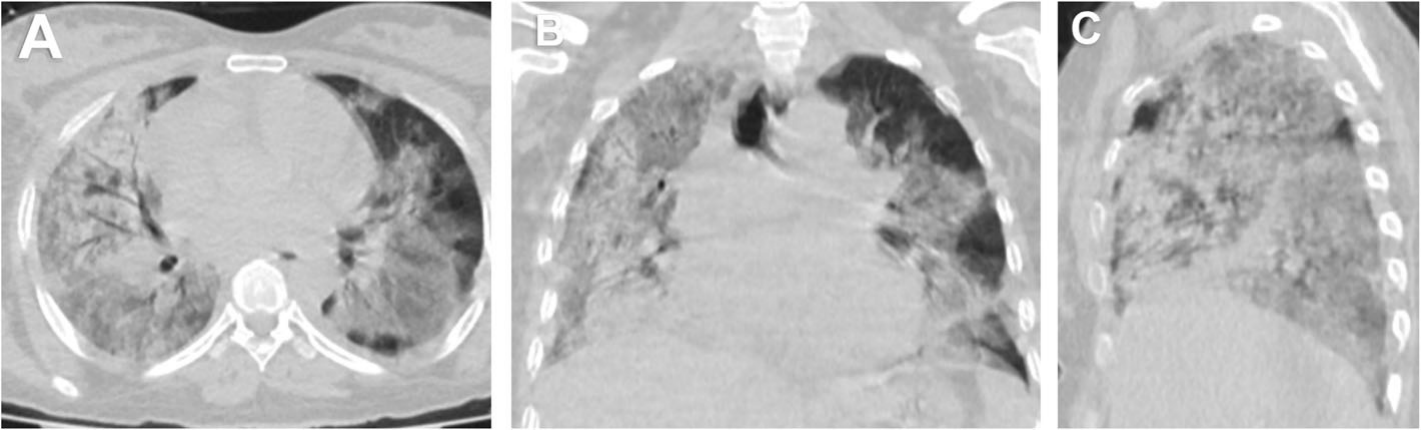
A 30-year-old female patient. A axial, B coronal, and C sagittal chest CT images show bilateral diffuse ground-glass opacities and consolidation with extensive pulmonary involvement (> 75%) corresponds to current chest CT score 22 and simple chest CT score 5 (extensive affection). The patient died on the fifth day
